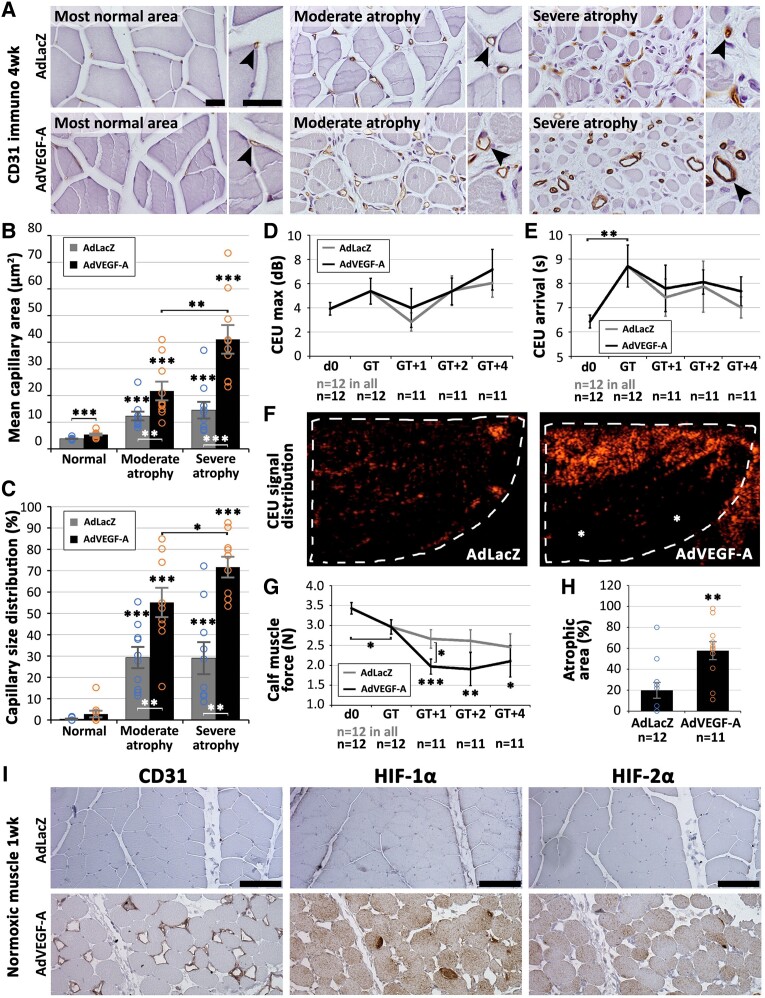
Capillary enlargement by AdVEGF-A under low arterial driving pressure aggravates ischaemic tissue damage. (*A*) Structural transformation of muscle capillaries (arrowheads) along worsening of myofibre atrophy seen in CD31 (brown) immunostanings 4 weeks in AdLacZ control transduced muscles and further capillary enlargement and arterialization induced by AdVEGF-A. Increased (*B*) mean capillary area and (*C*) capillary size distribution were quantified along worsening of myofibre atrophy. (*D*) Mean contrast-enhanced ultrasound (CEU) signal intensity did not significantly differ between gene transfer groups or as compared to normal (d0) values. (*E*) Mean CEU arrival time was significantly increased after the ischaemia operation but did not differ between the gene transfer groups. (*F*) Contrast-enhanced ultrasound signal (spekles) distribution in the transduced muscles (dotted line) was uneven after AdVEGF-A gene transfer leaving parts of the muscle unperfused (asterisks). (*G*) Calf muscle force production was reduced by the ischaemia operation at the time of gene transfer and further decreased after AdVEGF-A gene transfer. (*H*) Area of atrophic muscle damage increased after AdVEGF-A gene transfer. (*I*) Rounding, occasional atrophy, and increased expression of Hif-1a and Hif-2a of myofibres 1 week after AdVEGF-A gene transfer to healthy rabbit skeletal muscle as compared to changes in respective AdLacZ control. CEU, contrast-enhanced ultrasound; d0, baseline; GT, gene transfer; GT + X, X weeks after GT. Scale bar in main images 50μm, inset 25μm. P* < .05, *P* < .01, P* < .001
